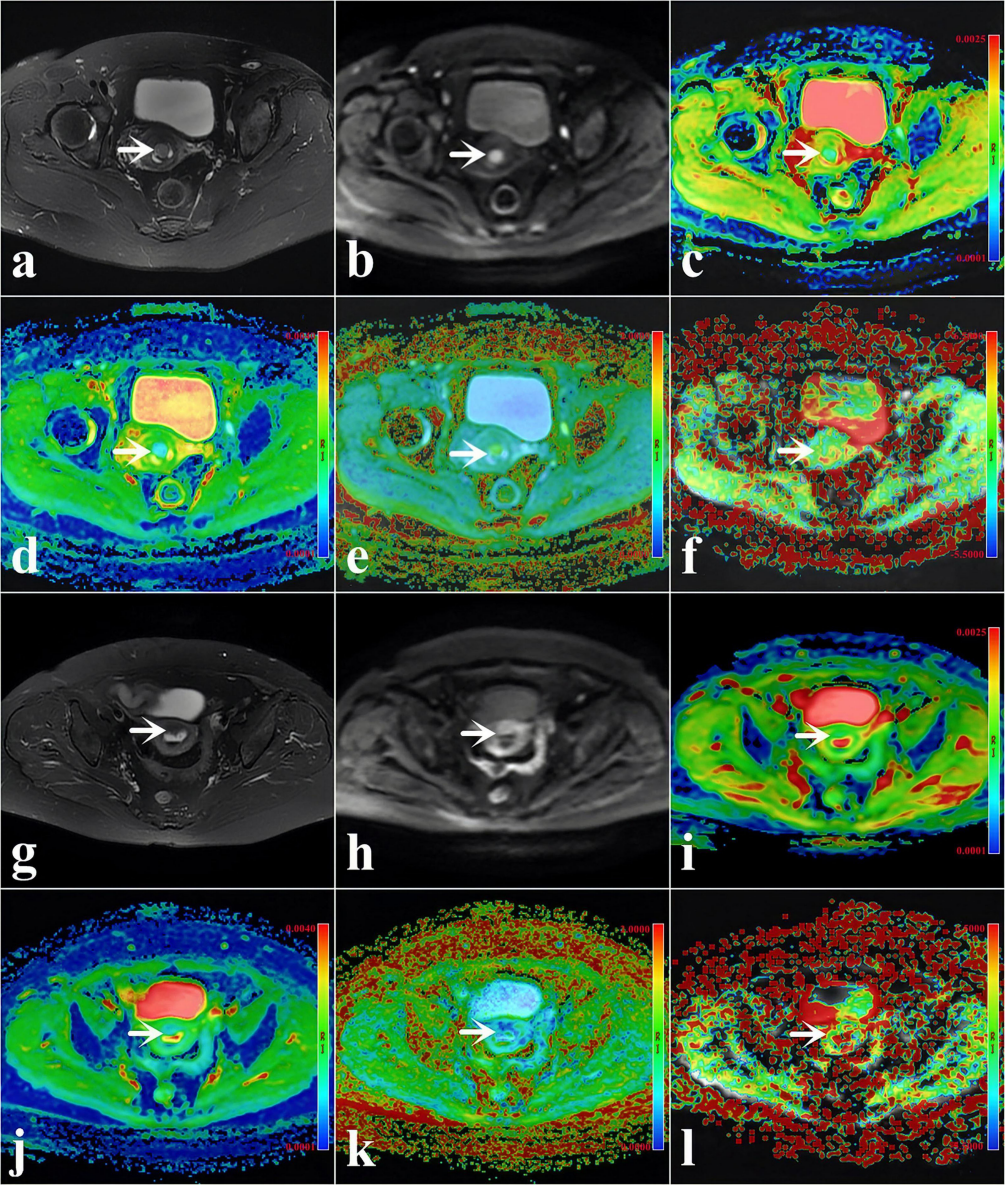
(A–F) A 48-year-old woman with low-risk endometrial cancer (EC) [*arrowheads*, endometrioid type; grade 1, stage IA, lymphovascular space invasion (LVSI)-negative]. (G–L) A 61-year-old woman with high-intermediate-risk EC (*arrowheads*, endometrioid type; grade 3, stage IA, LVSI-positive). (A, G) T2-weighted imaging (T2WI) maps (fat suppression). (B, H) Diffusion-weighted imaging (DWI) original maps (*b* = 1,000 s/mm^2^). (C, I) Pseudo-colored maps of the apparent diffusion coefficient (ADC). (D, J) Pseudo-colored maps of the mean diffusivity (MD). (E, K) Pseudo-colored maps of the mean kurtosis (MK). (F, L) Pseudo-colored maps of the magnetization transfer ratio asymmetry (MTRasym, at 3.5 ppm).
